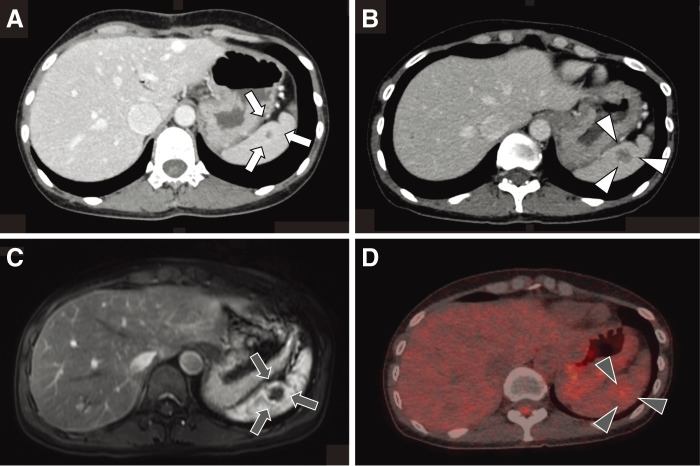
Imaging findings of a progressively enlarging splenic mass following a Whipple procedure. CE-CT performed 6 months after the Whipple procedure revealed a low-density splenic SOL measuring approximately 10 mm in diameter (white arrows) (A). At 12 months postoperatively, follow-up CE-CT showed that the lesion had increased in size to 14 mm (white arrowheads) (B). CE-MRI demonstrated a ring-enhancing lesion measuring 18 mm (gray arrows) (C). FDG-PET revealed FDG uptake within the mass, with a maximum standardized uptake value of 4.24 (gray arrowheads) (D). CE-CT, contrast-enhanced CT; FDG, fluorodeoxyglucose; SOL, space-occupying lesion
